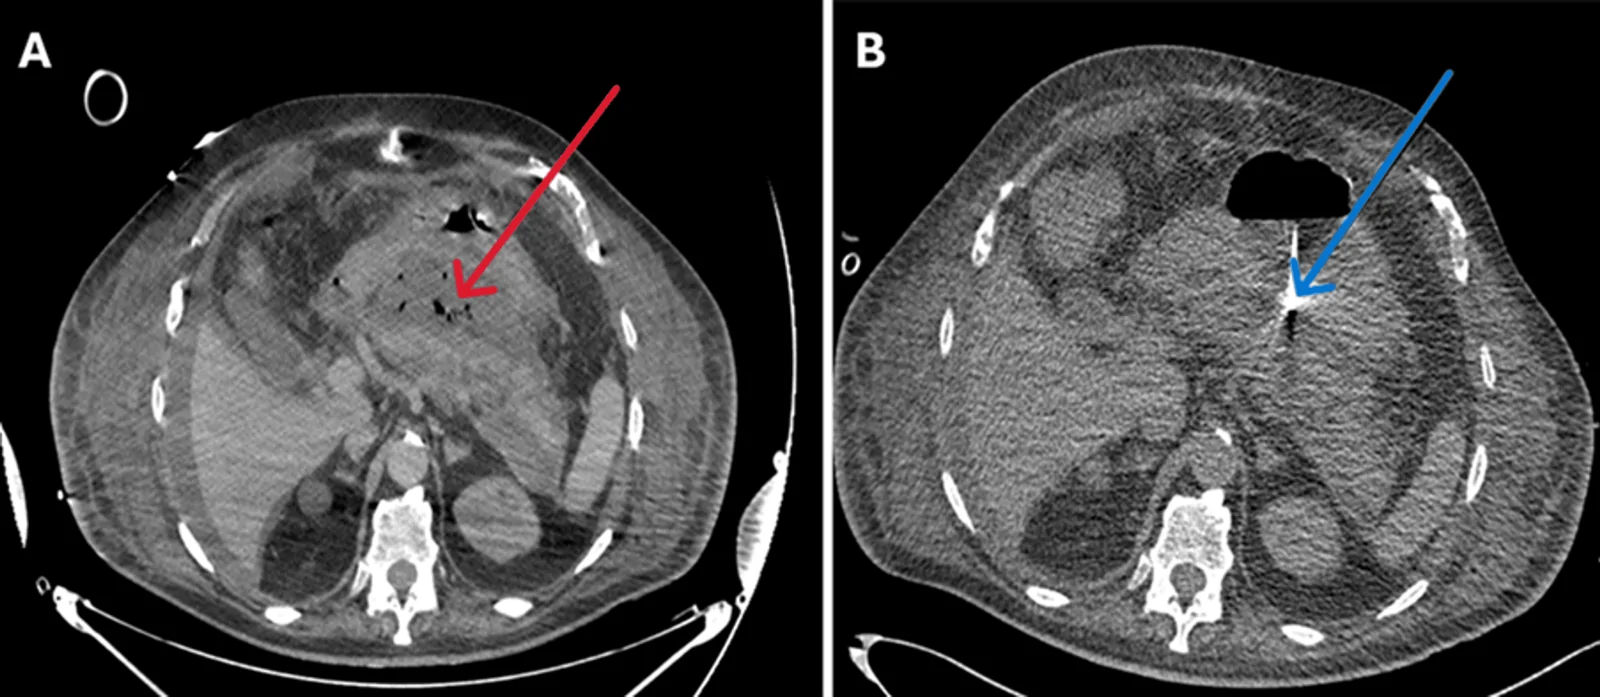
CT assessment of infected pancreatic necrosis before and after percutaneous drainage. (A) Preprocedural axial contrast-enhanced CT scan showing a heterogeneous peripancreatic collection with foci of gas (red arrow), consistent with infected pancreatic necrosis. (B) Postprocedural scan confirming the correct placement of the percutaneous drainage catheter (blue arrow) within the collection, with visible reduction in the collection volume.
